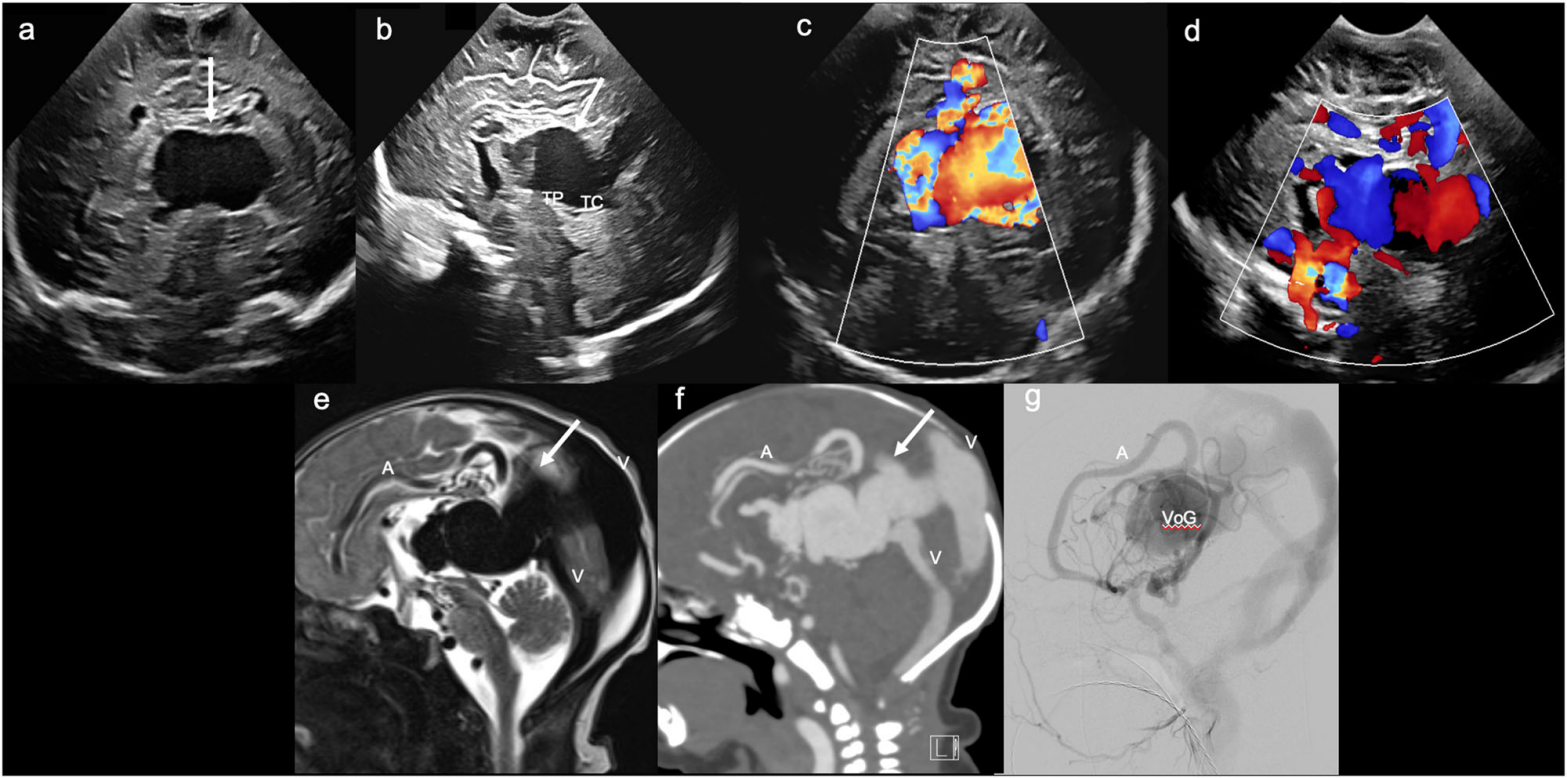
Vein of Galen aneurysmal malformation. Day 1 ultrasound in a term neonate with high-output cardiac failure. a Coronal image demonstrates a central, ovoid, anechoic mass (solid arrow). b Midline sagittal image confirms that the anechoic mass (solid arrow) is centred on the pineal recess, lying posterior to the tectal plate (TP) and superior to the tentorium cerebelli (TC). c Coronal and (d) midline sagittal colour Doppler images demonstrate marked vascularity within the sac, with aliasing artefact suggesting turbulent flow. e Midline sagittal T2WI and (f) contrast-enhanced CT demonstrate a vascular mass, with T2 low signal flow voids (e) and avid contrast enhancement (f). Large arterial feeders (A) and draining venous channels (V) are evident. g Midline sagittal digital subtraction angiography (DSA) demonstrates arteriovenous shunting of blood from a large anterior cerebral artery (A) to an enlarged/aneurysmal median prosencephalic vein (VoG)
